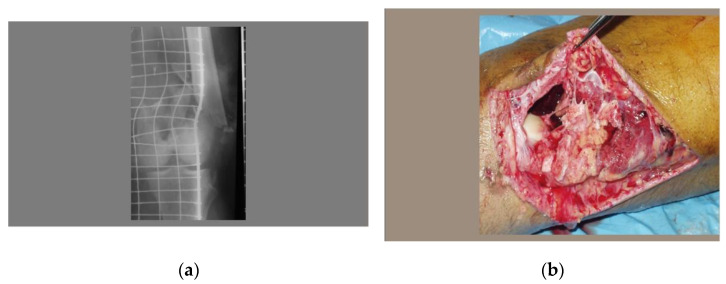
(a) X-ray of the supra and intercondylar multi-comminuted distal femur fracture; (b) open distal femur fracture—lateral view.
